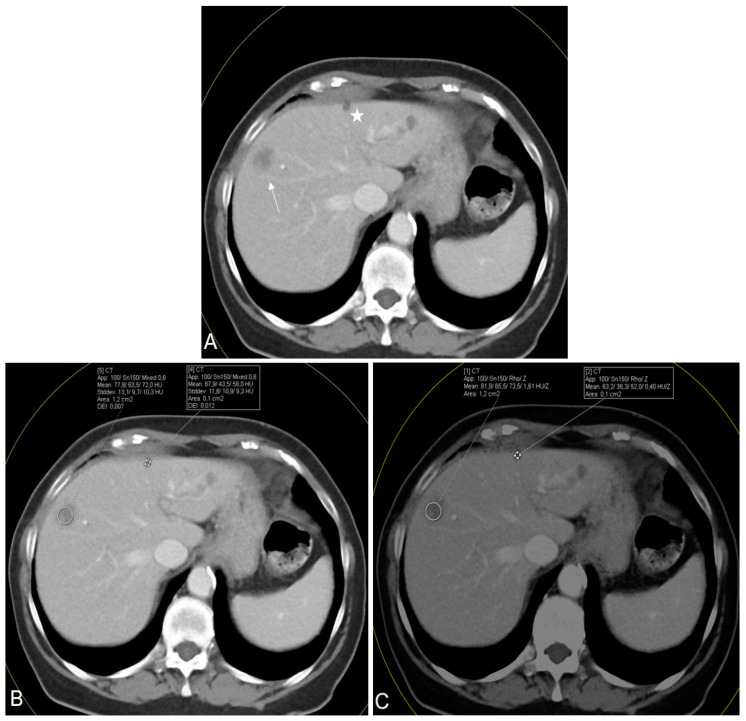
Axial contrast-enhanced CT scan in a 54-year-old female suffering from malignant melanoma. The scan showed multiple hypodense liver lesions in different segments, including a biopsy-confirmed liver metastasis in segment 8 (arrow) and an MRI-confirmed subcapsular liver cyst in segment 4 (star) (A). Conventional CT value measurements (B) demonstrated surprisingly high mean CT values for both lesions (72.0 and 58.0, respectively), while the mean Rho and Z values (C) showed greater differences for both lesions (Rho, 79.5 and 51.9; Z, 1.53 and 0.40, respectively) facilitating CT-based differentiation between metastases and cysts.
